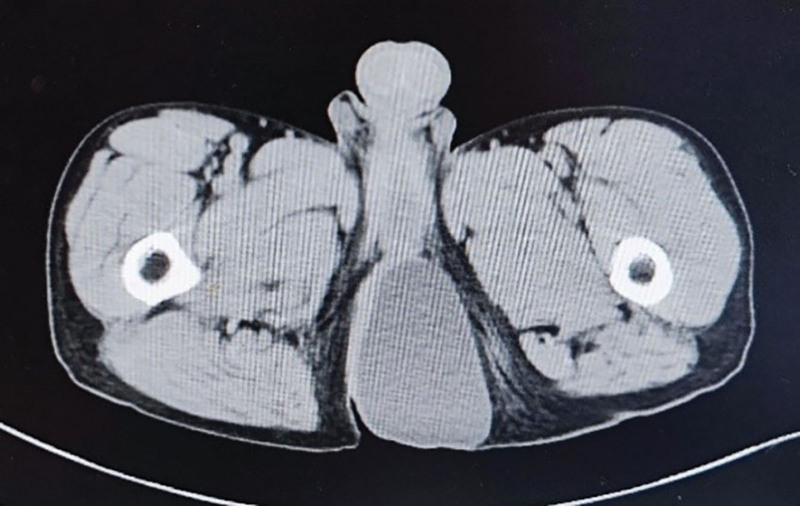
Axial CT image obtained through the pelvis demonstrates a circular cystic predominantly cystic solid shadow, the anus was compressed and shifted to the right. CT = computed tomography.
